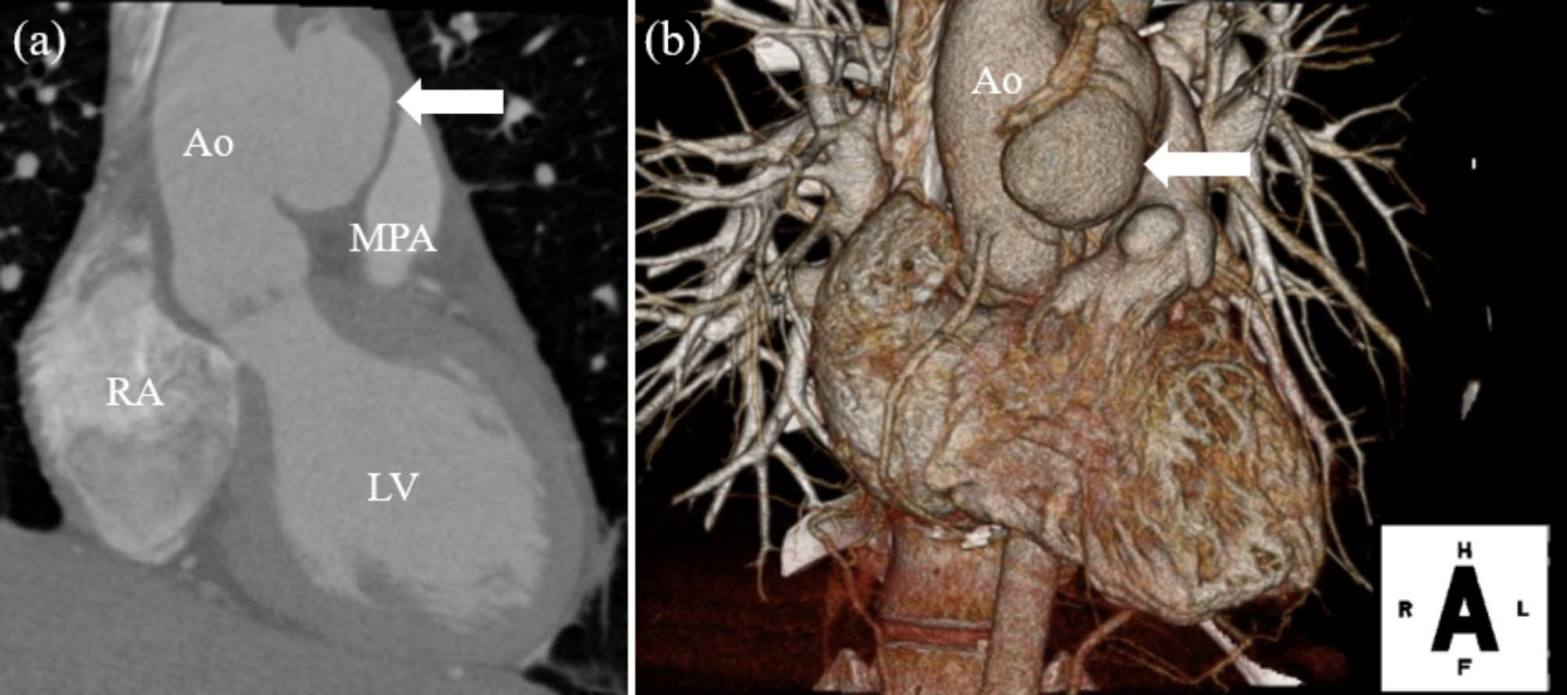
(a, b) Preoperative computed tomography images. CT showed a pseudoaneurysm of the ascending aorta (white arrow) Ao: aorta, MPA: main pulmonary artery, RA: right atrium, LV: left ventricle
